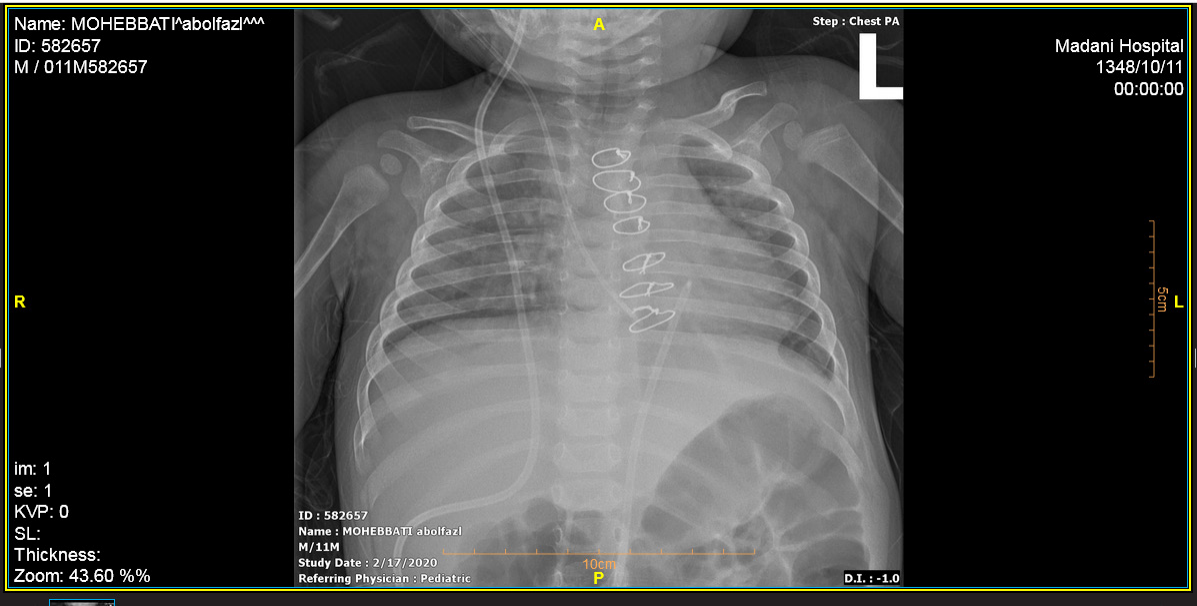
The patient planed for open-heart surgery and all the necessary arrangements were made.(Figure 3). Cardiopulmonary bypass was conducted under general anesthesia with a middle sternotomy technique and cannulation of the superior and inferior vena cava. The body was cooled to 30 °C and the cardiopulmonary solution was injected from the root of the heart aorta. In the right ventricle, a vegetation of 5 cm long was observed originating from the entrance point of the VP shunt and continuing up to the right ventricular outflow tract and the tricuspid valve. The vegetation was extracted and the tip of the VP shunt was directed back to the peritoneal cavity. The tricuspid valve was intact with appropriate function. The right ventricle and atrium were repaired and the patient was detached from cardiopulmonary bypass after warming up. Two drains were placed in the pleural space and one drain in the mediastinum and the sternum was closed. The patient was transferred to the intensive care unit (ICU) in stable condition and recovered after one day. Two weeks later, the patient was discharged in stable condition. Two weeks after discharge, the patient presented again with symptoms of vomiting, tachypnea, and lethargy. Chest X-ray and thoracic echocardiography revealed that the VP shunt was again migrated about 2 cm into the anterior mediastinum (Figures 4 and 5). The patient underwent reoperation under general anesthesia and the VP shunt was shortened and re-inserted into the peritoneal cavity. The patient was transferred to the ICU in stable condition and recovered after 6 hours. Antibiotic treatment was continued for six weeks and the patient was discharged in stable condition. In follow-up visits after two years, the VP shunt functioned well and no particular complication was observed.
